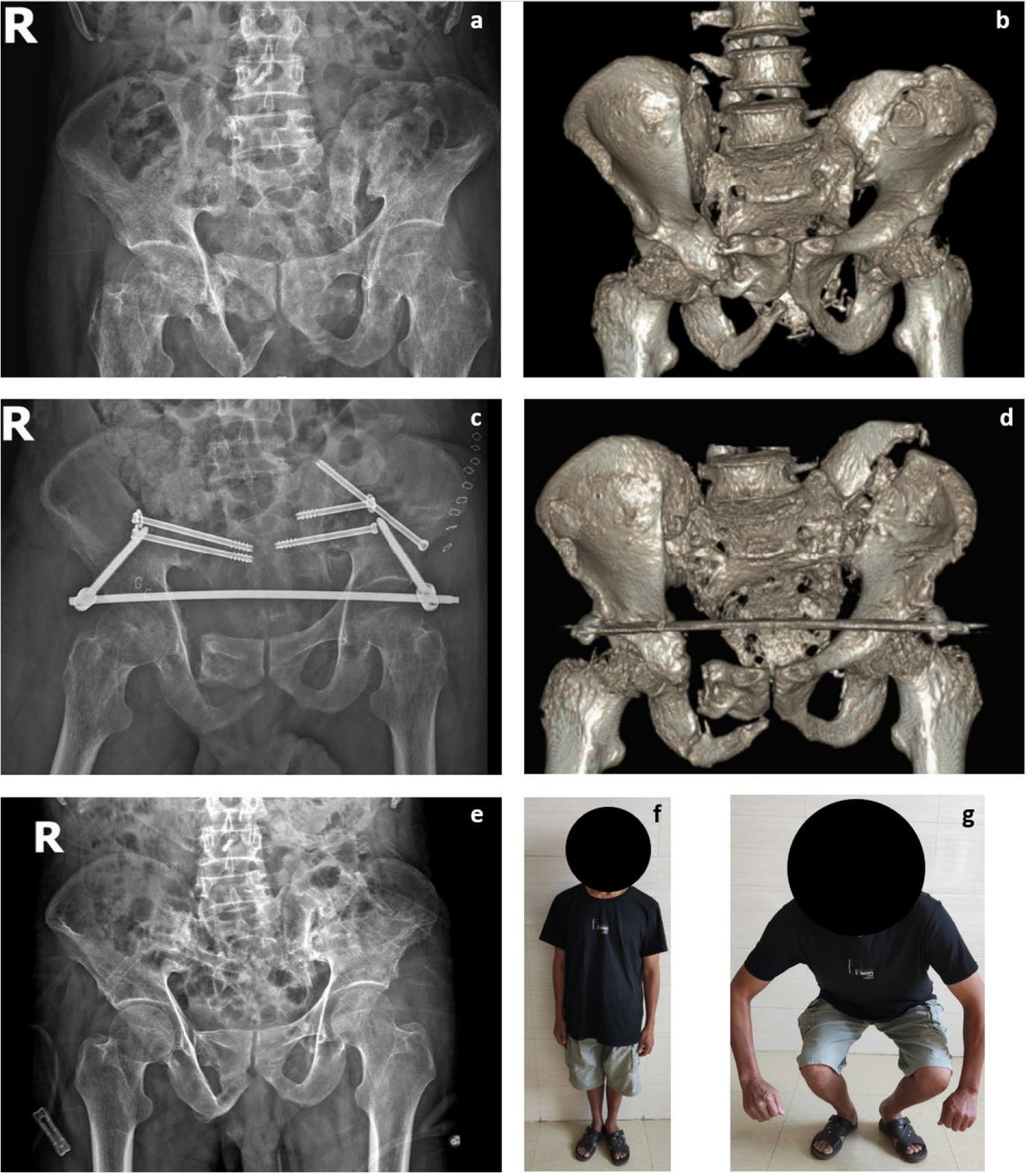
A case of complex deformity (male, 51 years old, injured by a car falling on him when repairing it under the chassis). The patient was sent to our institution 4 months after the injury, which was combined with pelvic infection and anal atresia after rectal colostomy, with a complaint of inability to stand and sit. Sacroiliac osteotomy was performed on both sides through the LRA. a, b Pre-operative AP X-ray view and 3D reconstruction of CT data. c, d Post-operative AP X-ray view and 3D reconstruction of CT data. e AP X-ray view at 2-year follow-up after removal of implants. f, g The patient’s quality of life was improved at the 2-year follow-up
